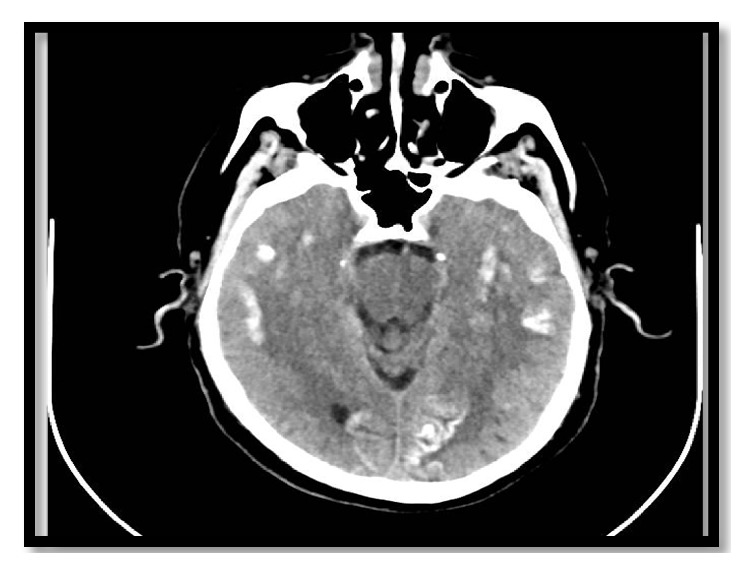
Plain CT of the brain at presentation to the hospital showing patchy and gyriform calcification in bilateral temporoparietal occipital corticosubcortical areas. Abbreviation: CT, computed tomography.
